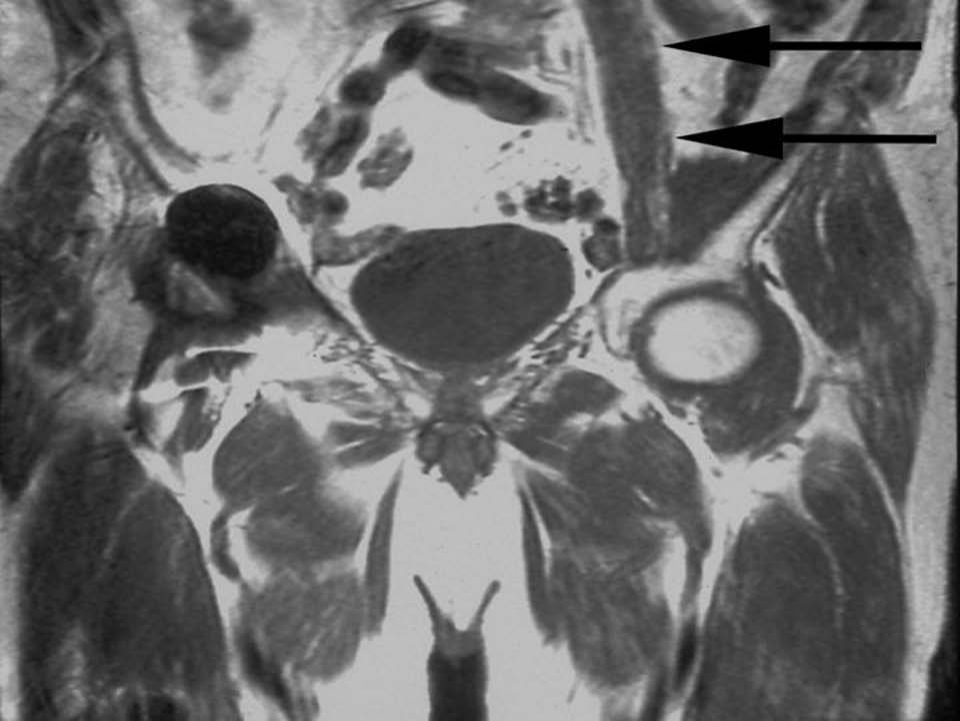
A coronal T1 MRI showing a normal ilio-psoas tendon on the left side (arrows) but its absence on the right side.
